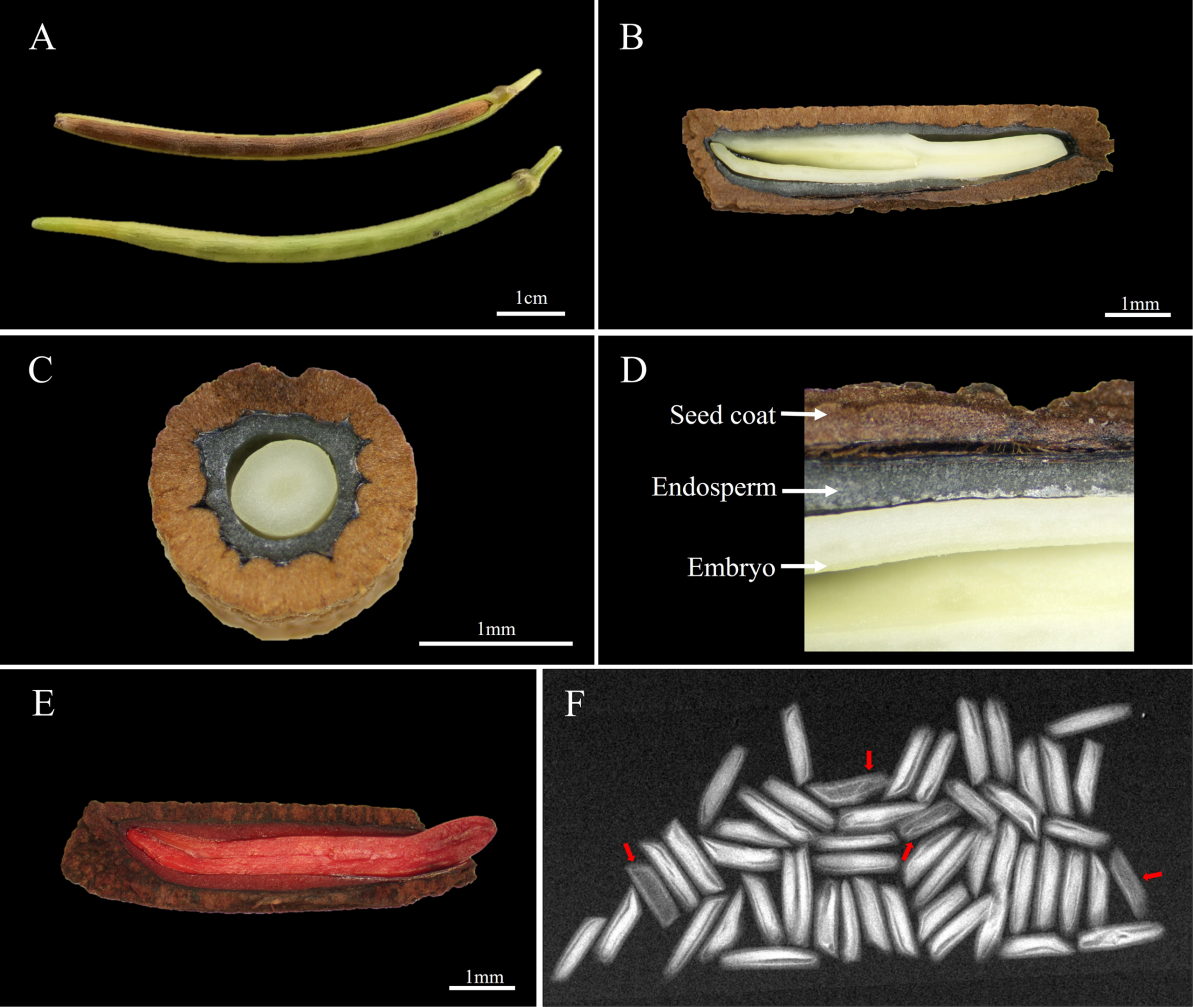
Morphological characteristics and viability of *Amsonia elliptica* seeds. (A), arrangement of the seeds in the follicle; (B), longitudinal section of seeds; (C), horizontal section of seeds; (D), the internal structure of seeds; (E), scarified seed after soaking in tetrazolium solution; (F), Fidelity of seeds confirmed by X-ray images. The red arrow represents empty seeds.
